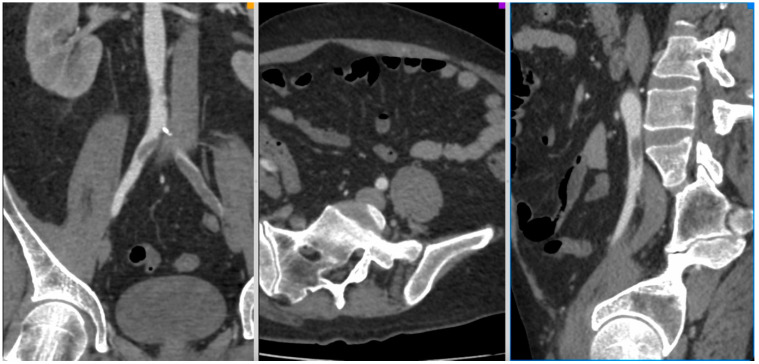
CT-Angio of a 42 yo male patient with aortic and iliac thrombosis with bilateral lower limb acute ischemia IIA Rutherford, 13 days after SARS-CoV-2 diagnosis.
